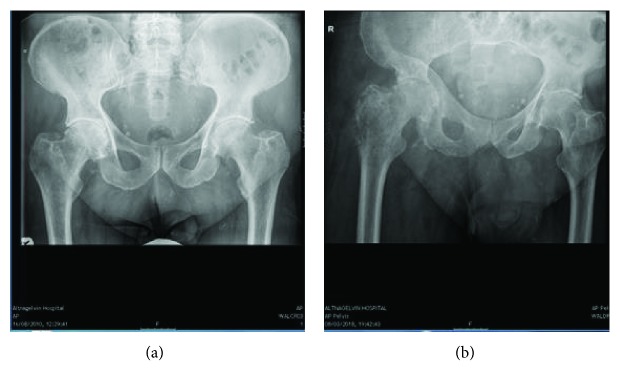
Plain film radiography compares pelvic radiographs from 2010 (a) and 2018 (b). On (a), the presence of moderately severe osteoarthritic change in the right hip is evident. On (b), there is a lytic lesion within the right femoral neck, intertrochanteric region, and proximal femoral metaphysis. Cortical breach with progressive sunburst periosteal reaction is evident.
